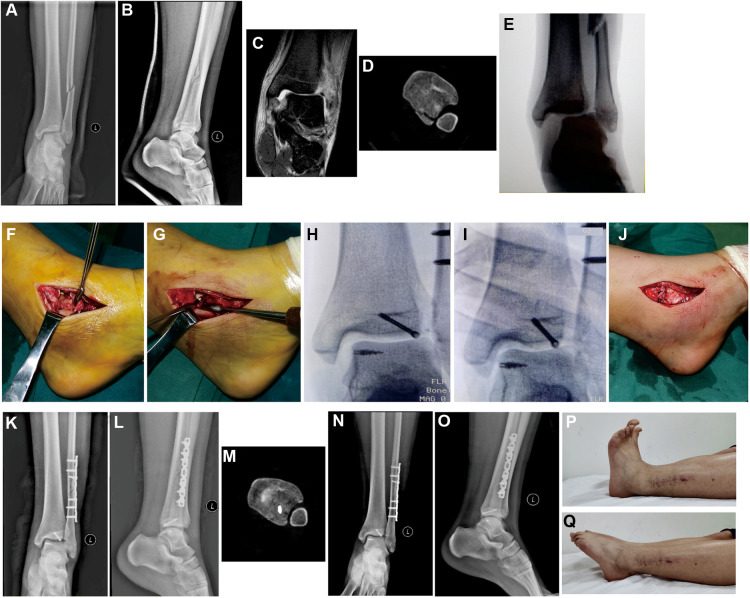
A Male patient diagnosed with Weber type C, Lauge–Hansen PER-IV, trimalleolar equivalent fracture treated with fibula ORIF and suture anchor deltoid ligament repair. Preoperative anteroposterior (A) and lateral view (B) showed that fibular fracture was above the tibiofibular syndesmosis, and the increased medial malleolus clear space was not obvious with plaster fixation. (C) MRI image (without plaster) indicated the deep deltoid ligament injury and increased medial malleolus clear space. (D) A CT scan image showed the posterior malleolus fracture and inferior tibiofibular syndesmotic disruption. (E) X-ray showed the increased medial malleolus clear space and inferior tibiofibular syndesmotic disruption with external rotation stress test. (F) Medial incision was used to show the injured superficial and deep deltoid ligament. (G) Suture anchor was placed on the astragalus insertion of deltoid ligament. (H) X-ray confirmed the position of suture anchor. (I) X-ray confirmed the reduction of medial malleolus clear space and inferior tibiofibular syndesmotic disruption after sutures were tightened. (J) Sutured superficial deltoid ligament and periosteum. Postoperative anteroposterior (K) and lateral view (L) of ankle. (M) Postoperative CT scan image to show the reduction of inferior tibiofibular syndesmotic disruption. One year follow-up x-ray examination, anteroposterior (N) and lateral view (O) showed the union of fracture without displacement. Ankle dorsal extension (P) and toe flexion (Q) were comparable with uninjured side at 1 year follow-up. PER , pronation-external rotation; ORIF, open reduction and internal fixation; MRI, magnetic resonance imaging; CT, computed tomography.
